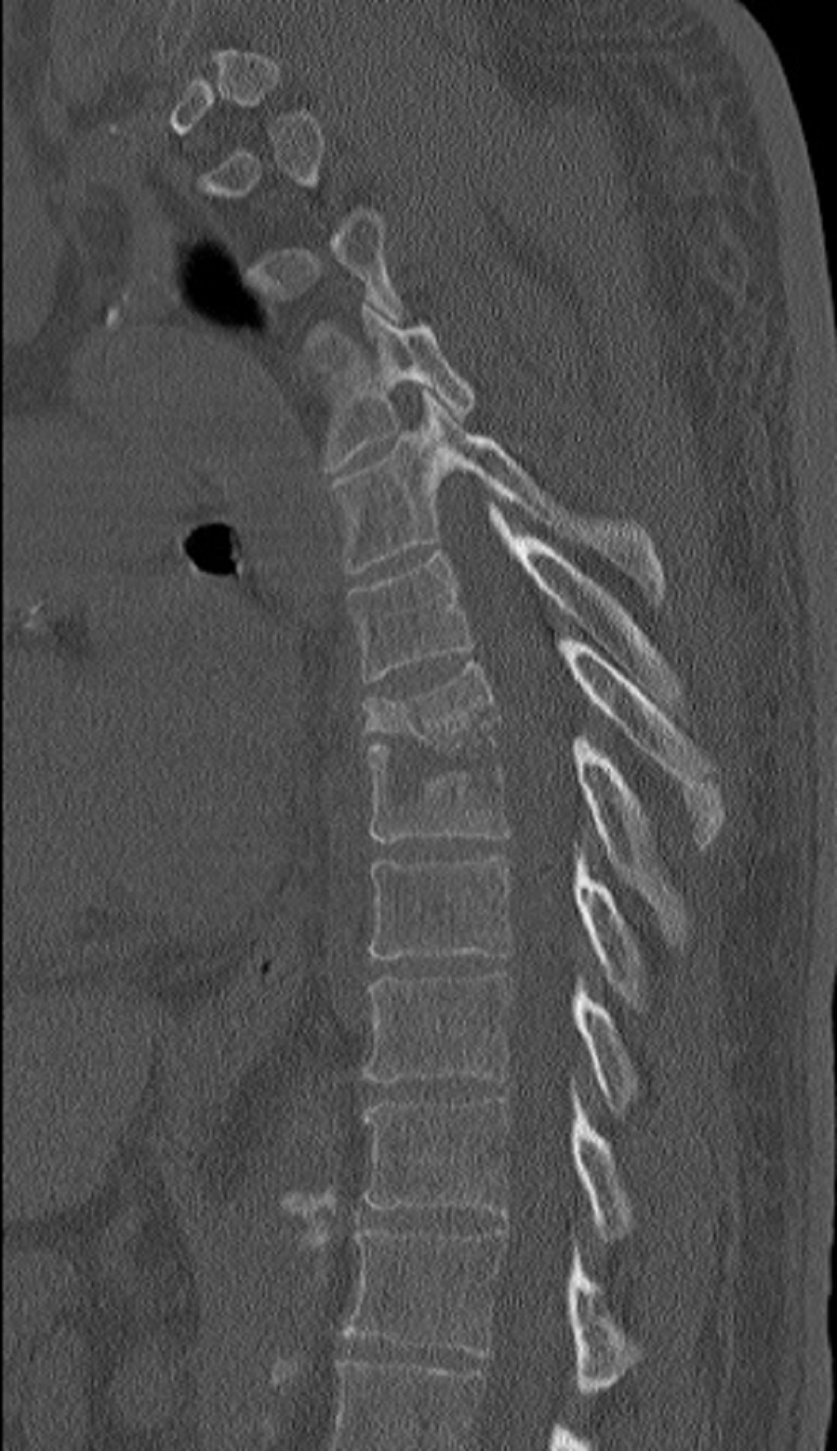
Preoperative sagittal CT demonstrating a T7-8 discitis and osteomyelitis
